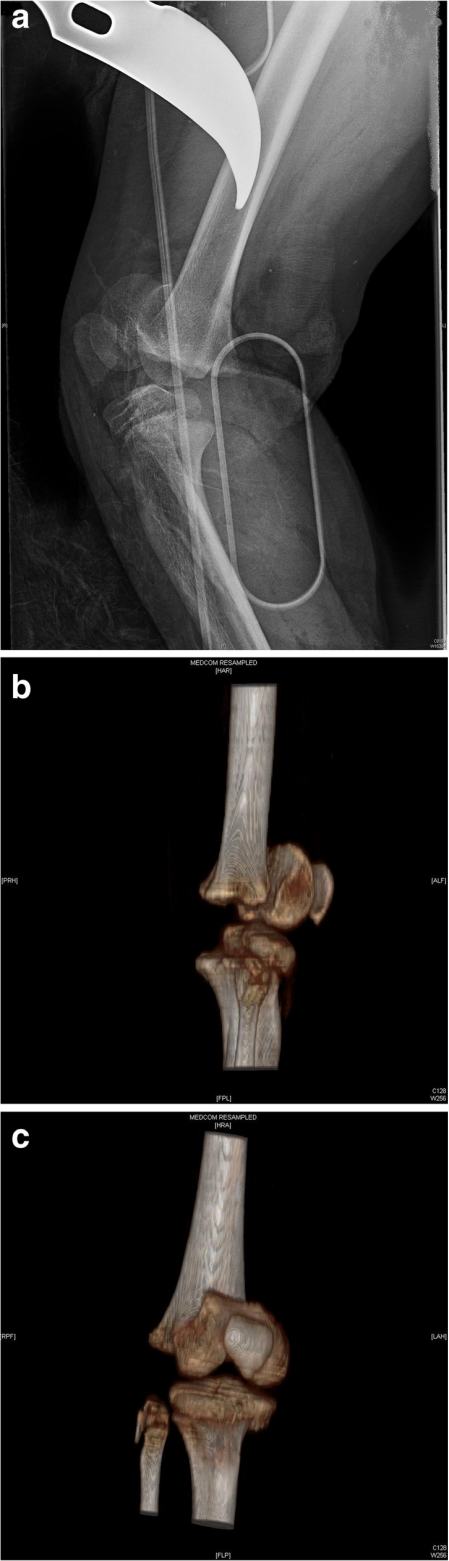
Preoperative radiological images demonstrating the severe right knee injury. (a) Lateral radiograph of the knee. (b) Sagittal computed tomography (CT) reconstruction showing the physeal fractures. (c) Coronal CT three dimensional reconstruction.
